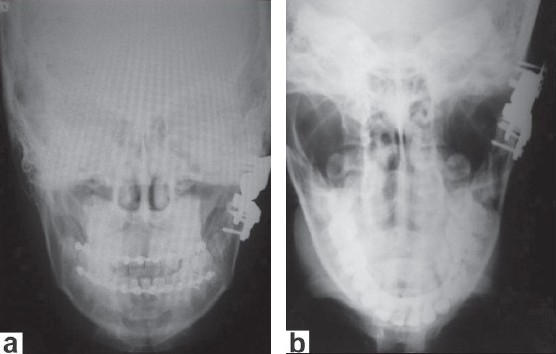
(a) Four-day postoperative X-ray shows that prosthesis is well fit; (b) Three-year postoperative X-ray shows that prosthesis is well in place and there are no signs of erosion at the skull base
